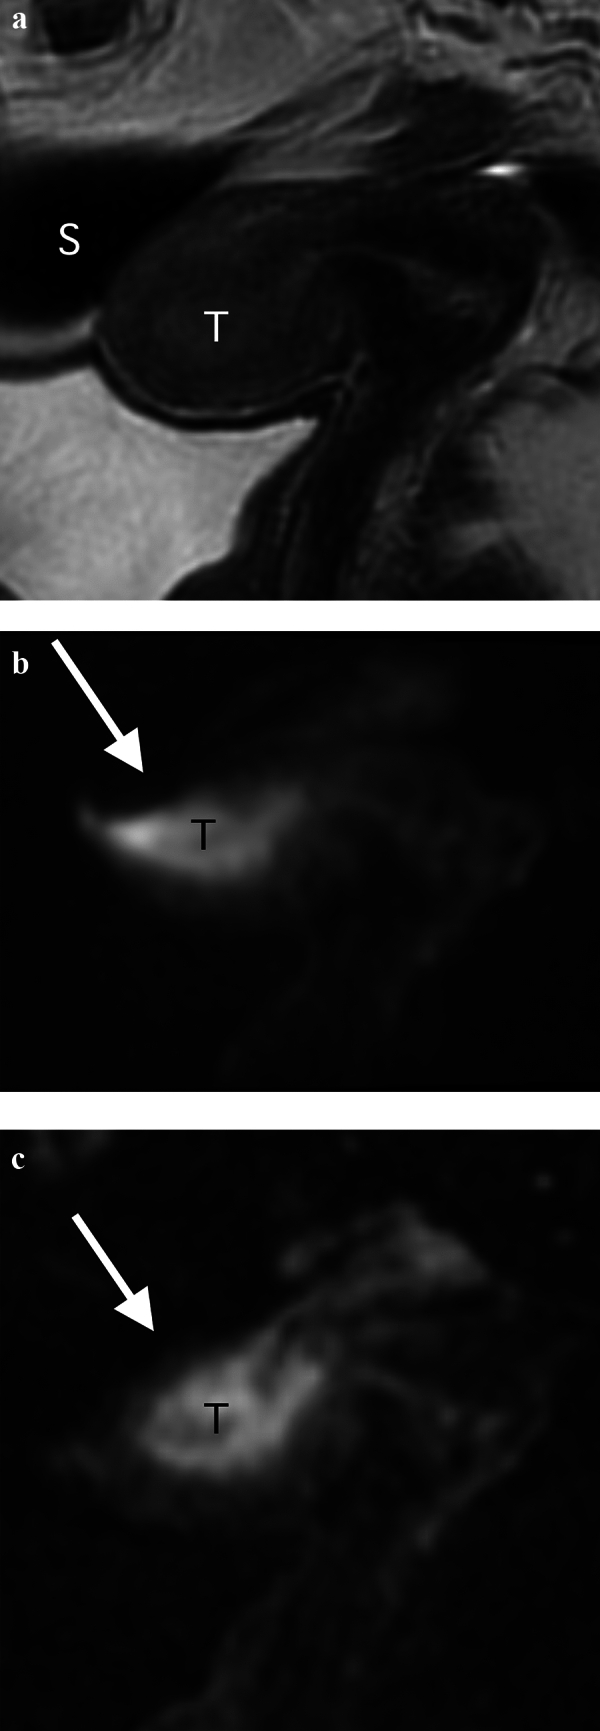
Images of a 66-year-old woman with endometrioid adenocarcinoma (Stage IB). a Parasagittal T2-weighted image shows a tumor (T) with intermediate signal intensity in the uterine cavity. There is gas-containing sigmoid colon (S) near the tumor. b Parasagittal reduced field-of-view diffusion-weighted image (rFOV-DWI) (*b* = 1000 s/mm^2^) shows the tumor (T) as an area of high signal intensity. Susceptibility artifacts are seen at air–tissue boundaries and the uterine structure is strongly distorted (arrow). c Parasagittal multiplexed sensitivity encoding diffusion-weighted image (MUSE-DWI) (*b* = 1000 s/mm^2^) shows the tumor (T) as an area of high signal intensity. MUSE-DWI depicts the tumor with significantly less susceptibility artifact compared to rFOV-DWI (arrow)
